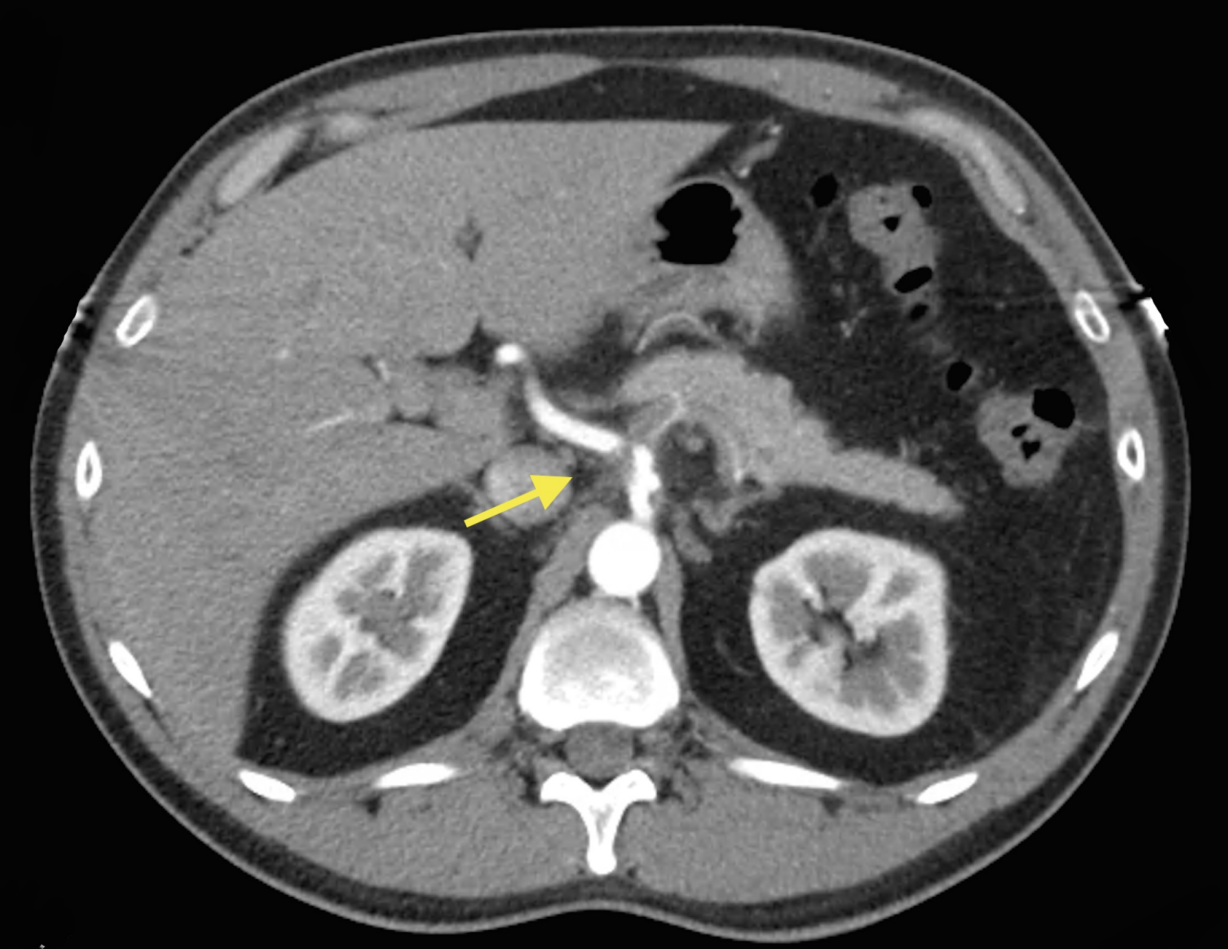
Computed tomography angiography (CTA) of the abdomen and pelvis depicting stenosis within the proximal celiac trunk of less than 50% with greater stenosis at the celiac bifurcation (arrow)
